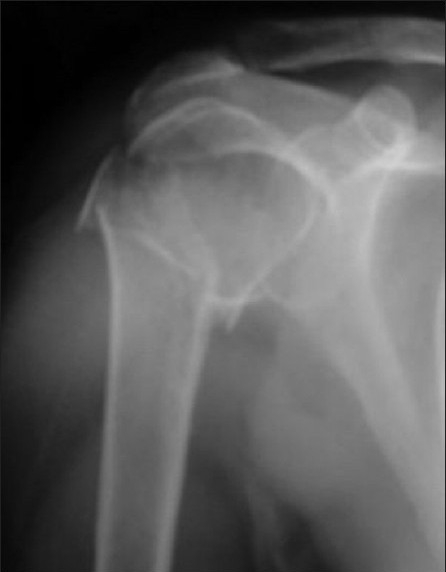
The radiographic appearance of the four-part fracture of the proximal humerus
